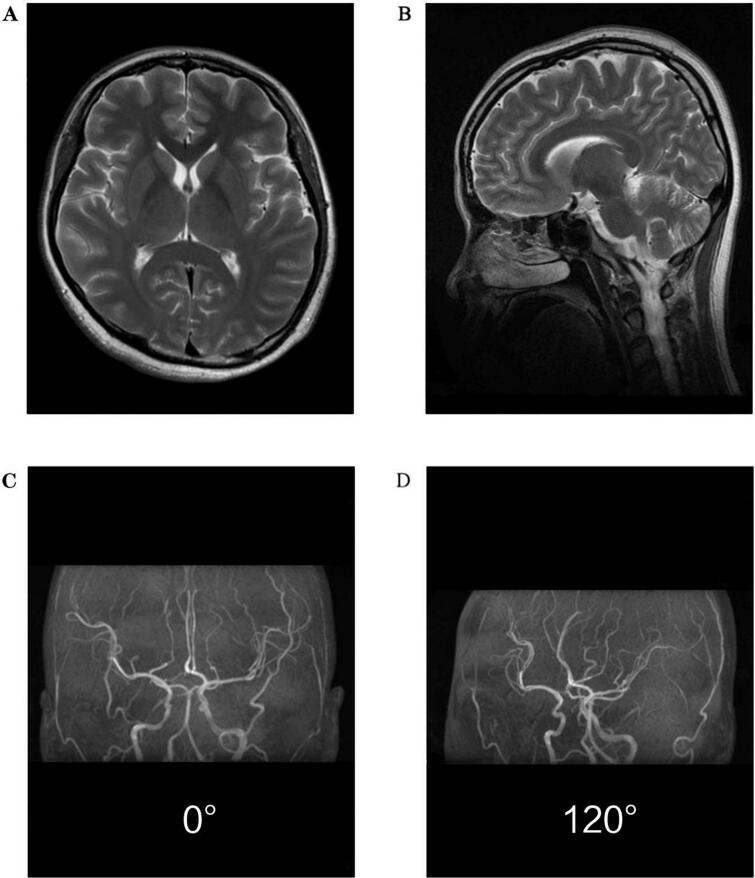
Neuroimaging findings in acute carbon monoxide poisoning. (A) Axial T2-weighted image (basal ganglia level): The head of the caudate nucleus, putamen, and posterior limb of the internal capsule demonstrate normal signal intensity without characteristic hyperintense lesions.(B) Sagittal T2-weighted image (central sulcus level): Uniform signal intensity is observed in the primary motor cortex and subcortical white matter, with no evidence of edema or necrosis.(C) Magnetic resonance angiography (MRA), anteroposterior projection (0°): The major arterial branches of the Circle of Willis (anterior cerebral artery [ACA], middle cerebral artery [MCA], and posterior communicating artery [PComA]) demonstrate normal course and patency.(D) MRA, oblique projection (120°): Focal luminal narrowing is noted in the A1 segment of the right ACA (arrow), consistent with congenital vascular developmental variation unrelated to acute toxic injury. Note: Despite severe clinical manifestations of carbon monoxide poisoning, no characteristic neuroimaging alterations were detected in susceptible gray matter predilection sites or cerebrovascular structures, contrasting distinctly with the observed brachial radiculopathy. This imaging-pathological dissociation suggests potential limitations of conventional MRI in detecting early neurotoxic damage.
